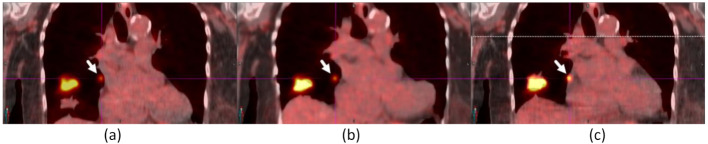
A 0.8-cm para-cardiac lymph node (indicated by an arrow) was registered in DDG CT (c), but mispositioned to the lung parenchyma in both baseline (a) and AIR (b). The SUV_max_ values for this lymph node were 4.76 (baseline), 5.01 (AIR), and 5.56 (DDG CT). Furthermore, a lung lesion located 3 cm above the diaphragm showed improved registration in both AIR and DDG CT. Its SUV_max_ values were 9.02 (baseline), 11.27 (AIR), and 10.64 (DDG CT)
